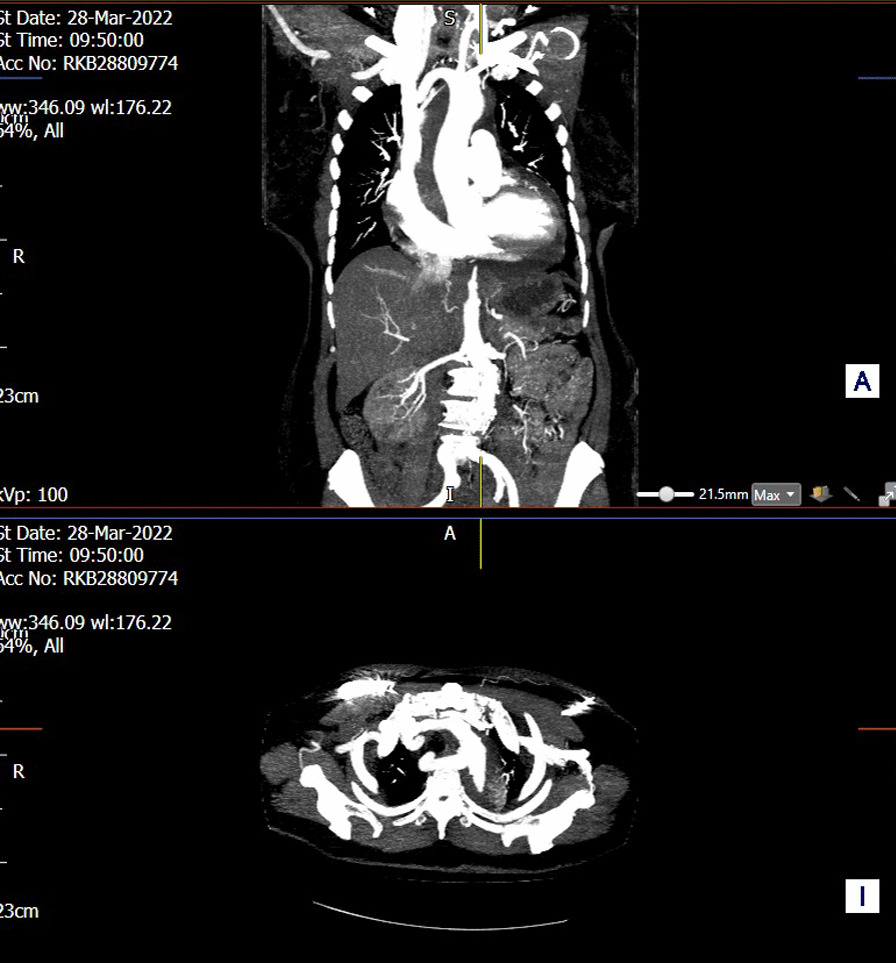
CT scan showing aberrant right subclavian artery arising from the distal arch coursing retro-tracheal forming a vascular ring and right common carotid artery taking an anomalous course with a common carotid trunk that was giving both carotids
